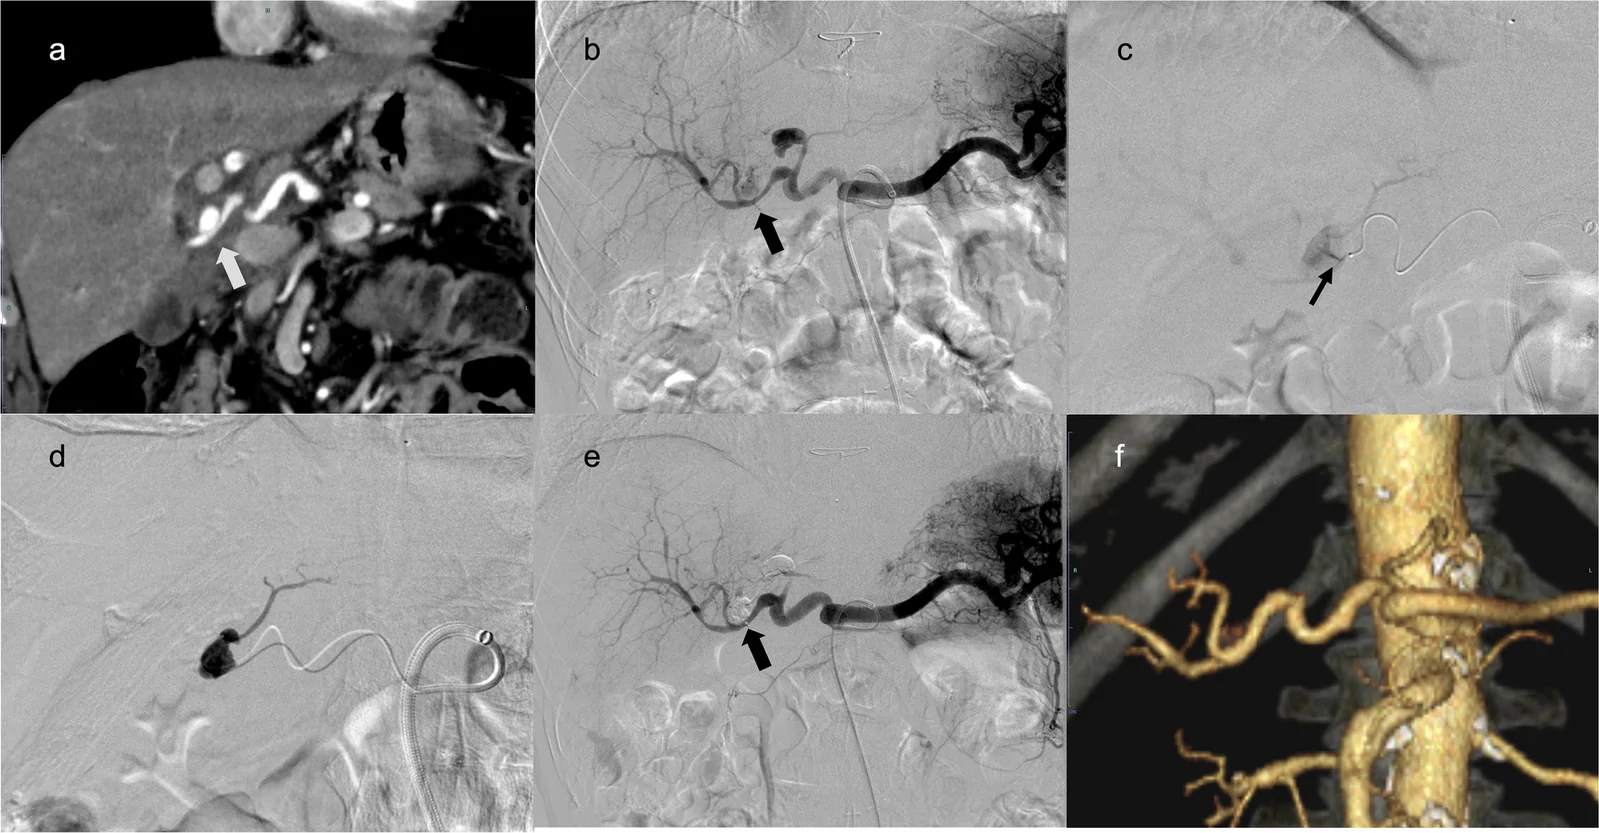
A 68-year-old man with a dissection extending from the common hepatic artery to the right and left hepatic arteries (Case 3). a Coronal reformatted contrast-enhanced computed tomography shows a dissecting aneurysm of the right hepatic artery (arrow). Soft-tissue attenuation surrounding the common hepatic artery suggests an intramural hematoma corresponding to a non-opacified false lumen. A dissecting aneurysm of the left hepatic artery is also visible. b Celiac arteriography shows the false lumen of the right hepatic arterial dissecting aneurysm (arrow), a dissecting aneurysm of the left hepatic artery, and smooth stenosis of the right hepatic artery, presumably caused by the intramural hematoma. c Contrast injection through a microcatheter positioned immediately proximal to the entry shows jet-like contrast inflow through the entry (arrow), clearly demonstrating its pinhole configuration. A caudate branch arising from the false lumen is also faintly opacified. d The microcatheter was advanced through the pinhole entry into the false lumen, followed by injection of the N-butyl cyanoacrylate–iodized oil mixture. No balloon was used because the entry was extremely small and NBCA reflux into the true lumen was considered unlikely. NBCA also entered a caudate branch arising from the false lumen. e Celiac arteriography immediately after treatment shows disappearance of false-lumen opacification. The dissecting aneurysm of the left hepatic artery had also been embolized with NBCA. f Three-dimensional volume-rendered image from contrast-enhanced computed tomography obtained 4 years later for surveillance of the thoracic and abdominal aortic dissections shows resolution of the dissecting aneurysms and disappearance of the hepatic arterial stenosis
